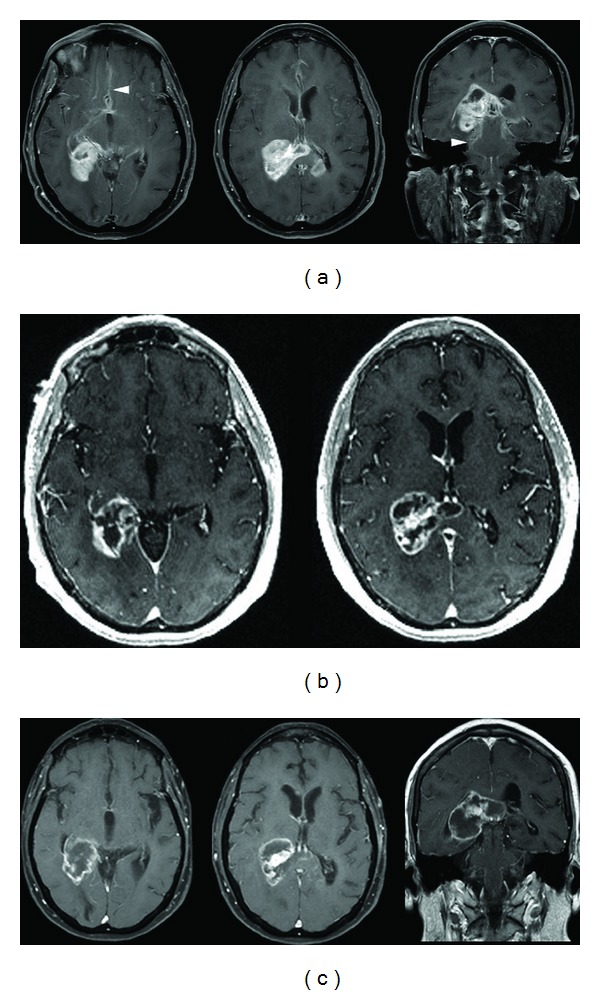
(a) Initial contrast-enhanced axial and coronal T1-weighted fast spin echo (FSE) sequence demonstrating an avidly enhancing temporoparietal mass in a 57-year-old female patient who presented with short-term memory loss, headaches, subtle left-sided weakness, and unsteady gait. There is enlargement of the splenium with nodular enhancement within the contralateral corpus callosum. Extensive areas of subependymal and leptomeningeal enhancement (arrowheads) are present. (b) Contrast-enhanced axial spoiled gradient recalled (SPGR) sequence demonstrating overall decreased enhancement with formation of centrally necrotic areas after 5 days of corticosteroid therapy. The patient's improved functional status and the radiographic regression of the mass suggested a diagnosis of lymphoma. (c) Axial and coronal T1-weighted, contrast-enhanced FSE image obtained two weeks later showing increased nodular enhancement along the inferior and medial margins of the dominant mass and evolution of the necrotic areas. These changes suggested a diagnosis of glioma.
